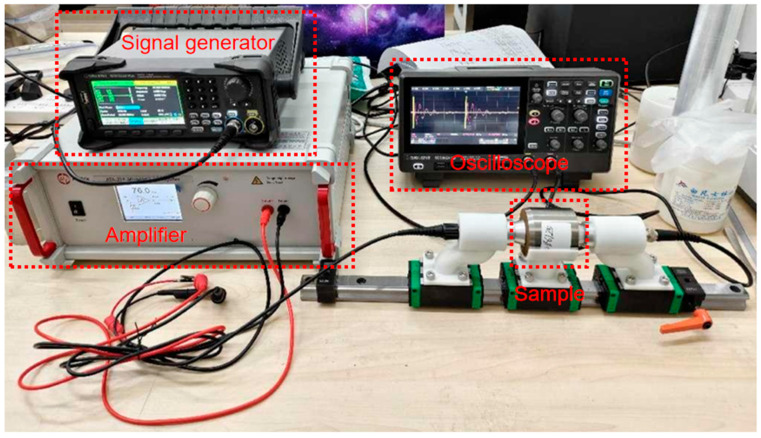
Equipment for ultrasound data acquisition.
